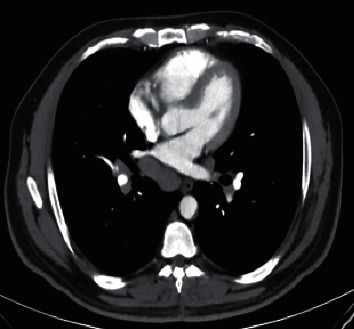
CT chest showing bilateral mediastinal and hilar adenopathy.
